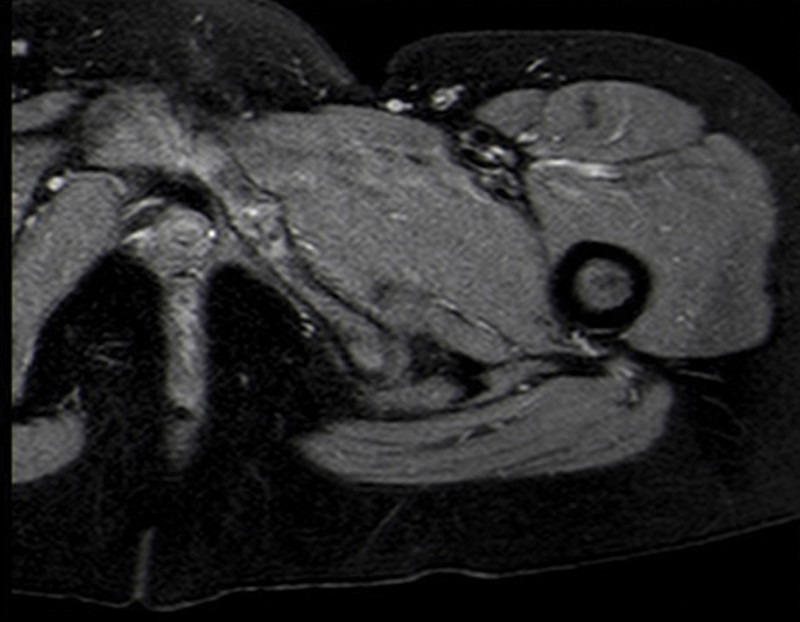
Magnetic resonance imaging of the pelvis, revealing edema at the level of the left ischiopubic synchondrosis.
